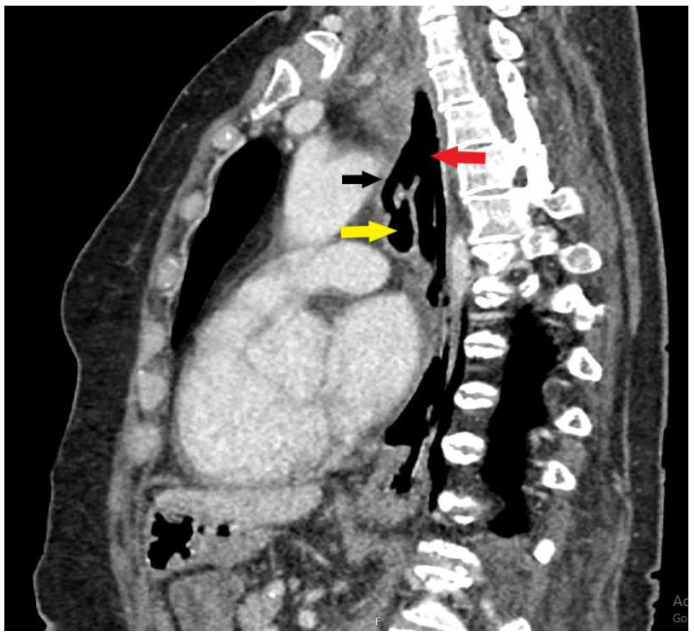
A sagittal CT scan shows interluminal communication (black arrow) between the lumen of the esophagus (red arrow) and the trachea (yellow arrow). There were also signs of aspiration pneumonia bilaterally and segmental pulmonary artery embolism (Figure 3).
